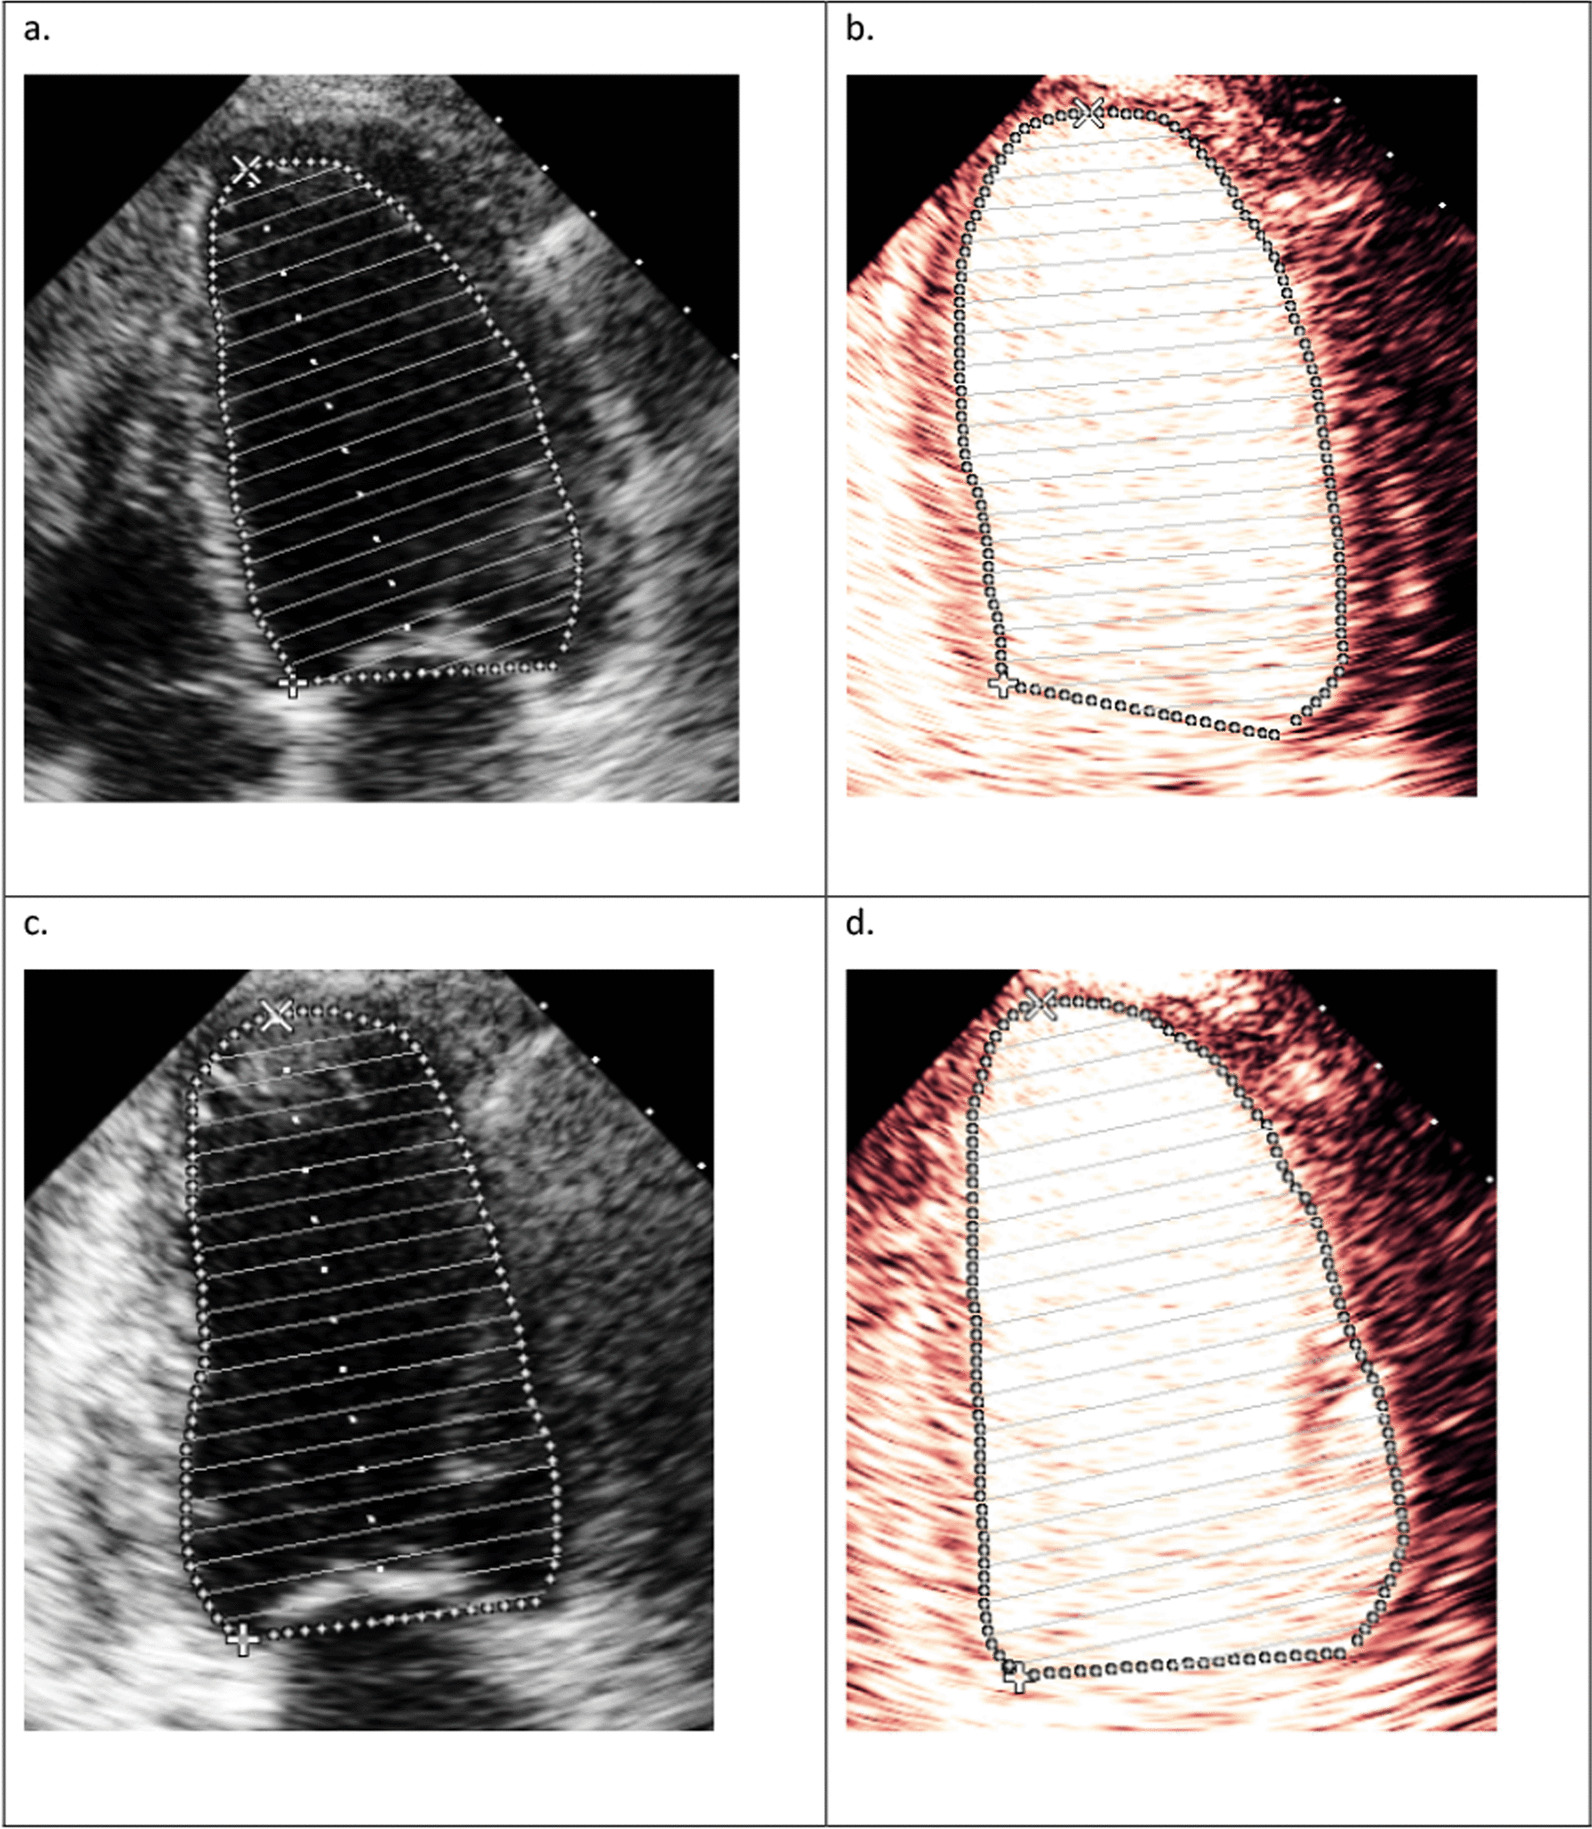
a Unenhanced apical 4 chamber with a diastolic LV volume of 103 ml. b Contrast enhanced apical 4 chamber in diastole measuring a LV volume of 114 ml. c Unenhanced apical 2 chamber measuring a diastolic volume of 105 ml. d Contrast enhanced apical 2 chamber in diastole measuring a LV volume of 127 ml
